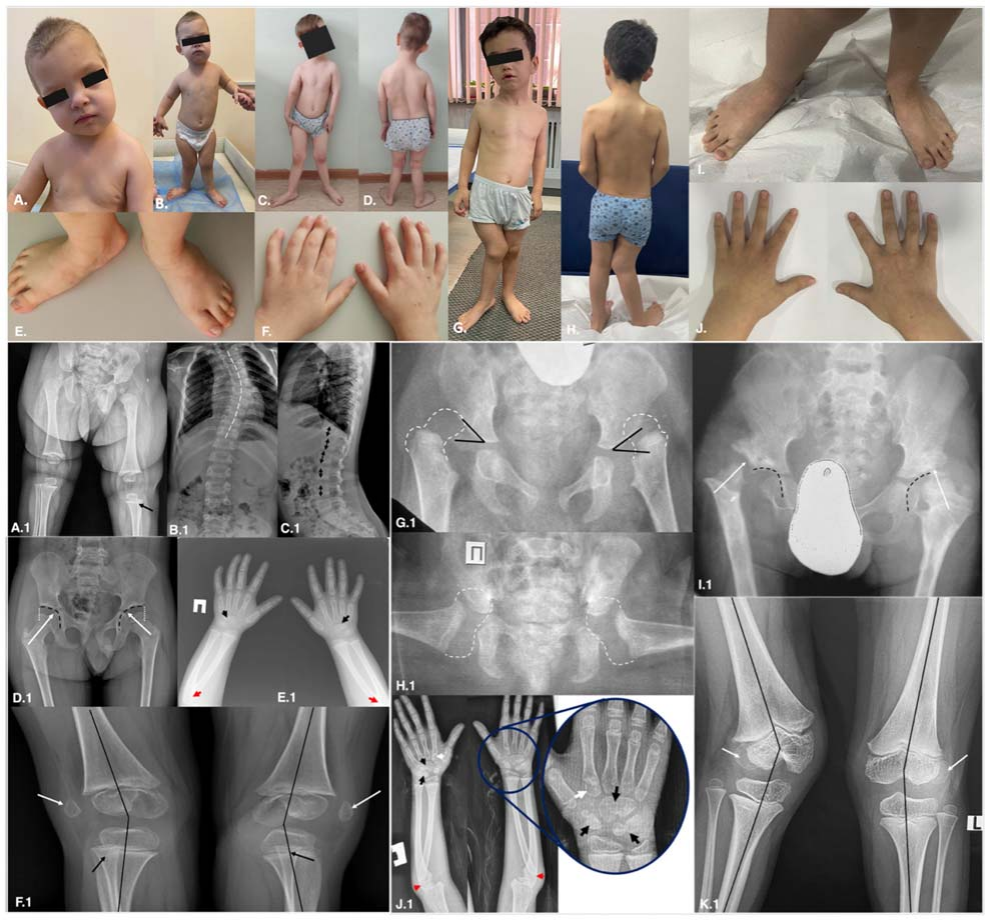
P1 phenotype at the age of 3.6 years (A, B) and at 5 years (C–F). P2 phenotype at the age of 7 years (G-J). Prominent forehead, hypertelorism, mild rhizomelic limb shortening, mild pectus excavatum, bilateral 5^th^ finger clinodactyly, genu valgum and mild partial cutaneous syndactyly of 2^nd^ and 3^rd^ toes were observed in both probands. P1 radiological findings at 10 months (A.1) and 5 years (B.1–F.1). P2 radiographs at 1 year (G.1;H.1) and at 6 years (I.1–K.1). (A.1) Normally centered hip joints with mild acetabular dysplasia, rotational subluxation of the knee presented with dorsally displaced proximal fibula (black arrow). (B.1;C.1) Severe thoracic scoliosis (white broken line), ‘tall' vertebral bodies (black arrows). (D.1) Normally centered hip joints with mild acetabular dysplasia (white arrows) with mild undercoverage of the femoral heads (white dotted lines). (E.1) Radial head dislocations (red arrows), multiple fusions of the carpal bones (black arrows). (F.1) Valgus knee deformity (black lines), lateral dislocation of the patella (white arrows), lateral and rotational subluxation of the tibiae. (G.1) Bilateral hip dislocation, dysplastic acetabulae (acetabular index 45° marked with the black lines). (H.1) Frog-leg position under the general anesthesia during an attempt of close reduction demonstrates irreducibility of the dislocations; non-ossified proximal femora marked with white broken lines. (I.1) Radiograph of the hips after surgery: residual hip dislocation, deformed proximal femoral neck and head oriented onto the supraacetabular region (white arrows); acetabular cavities are empty (marked with black broken lines). (J.1) Radial head dislocations (red arrows), multiple fusions of the carpal bones (black arrows), abnormal ossification pattern of the second metacarpals – pseudoepiphysis of the base of the second metacarpal bones (white arrows). (K.1) Valgus knee deformity (black lines); lateral dislocation of the patella (white arrows), lateral and rotational subluxation of the tibiae.
